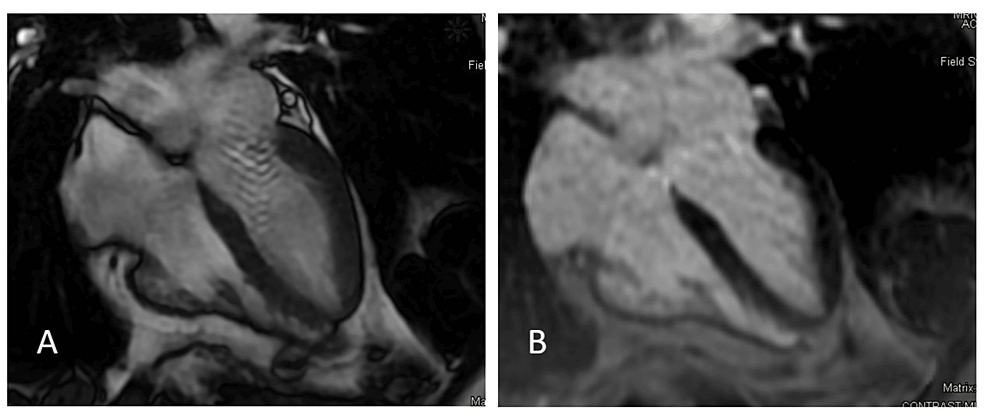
Cardiac MRI through the four-chamber view. Panel A shows a steady-state free precession frame in systole demonstrating normal left ventricular systolic function. Panel B demonstrates the corresponding phase-sensitive inversion recovery sequence showing a normal left ventricle with no evidence of late gadolinium enhancement
